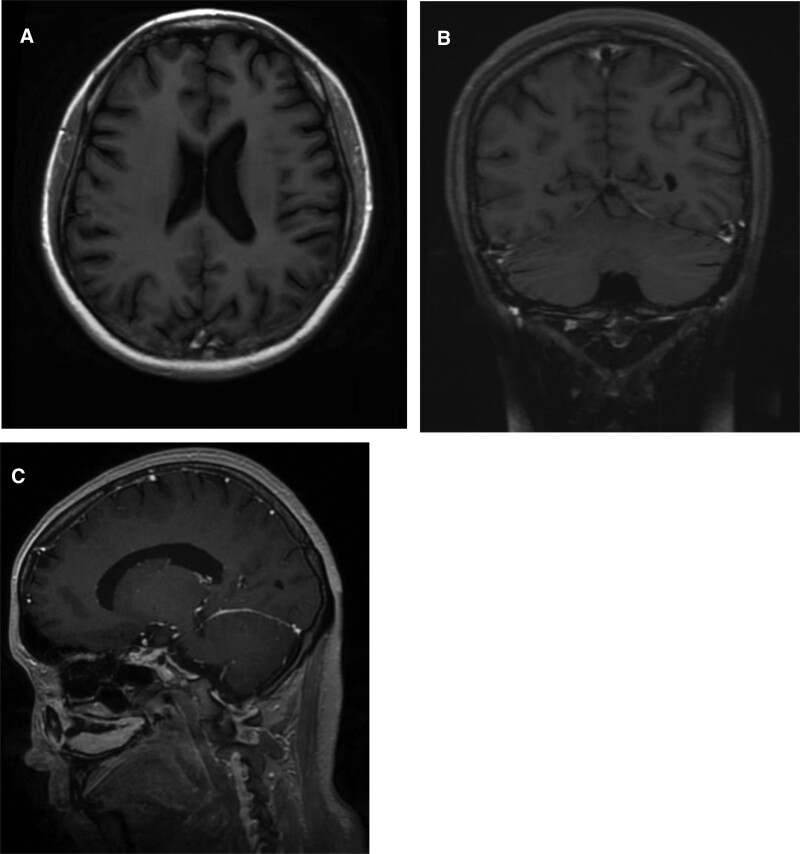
A, B and C show the MRI axial, coronal and sagittal enhancement images after antifungal treatment on the 19th day of admission, with a small amount of enhancement in the cerebellar sulcus and smooth margins. MRI = magnetic resonance imaging.
